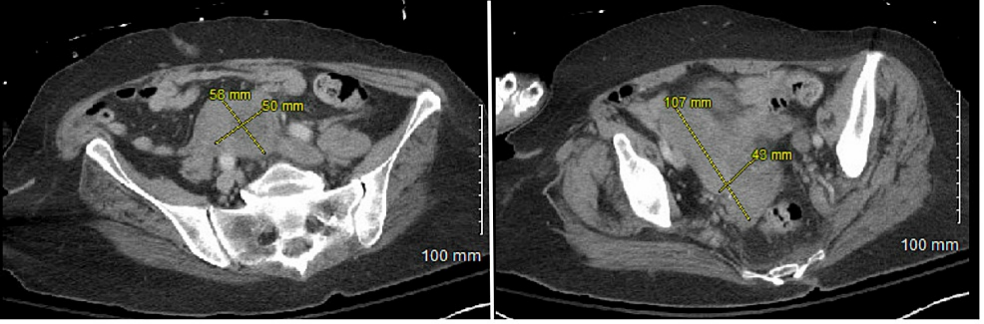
CT of the chest, abdomen, and pelvis showing findings compatible with worsening lymphatomatous disease, with right pelvic and mesenteric lymphadenopathy.
